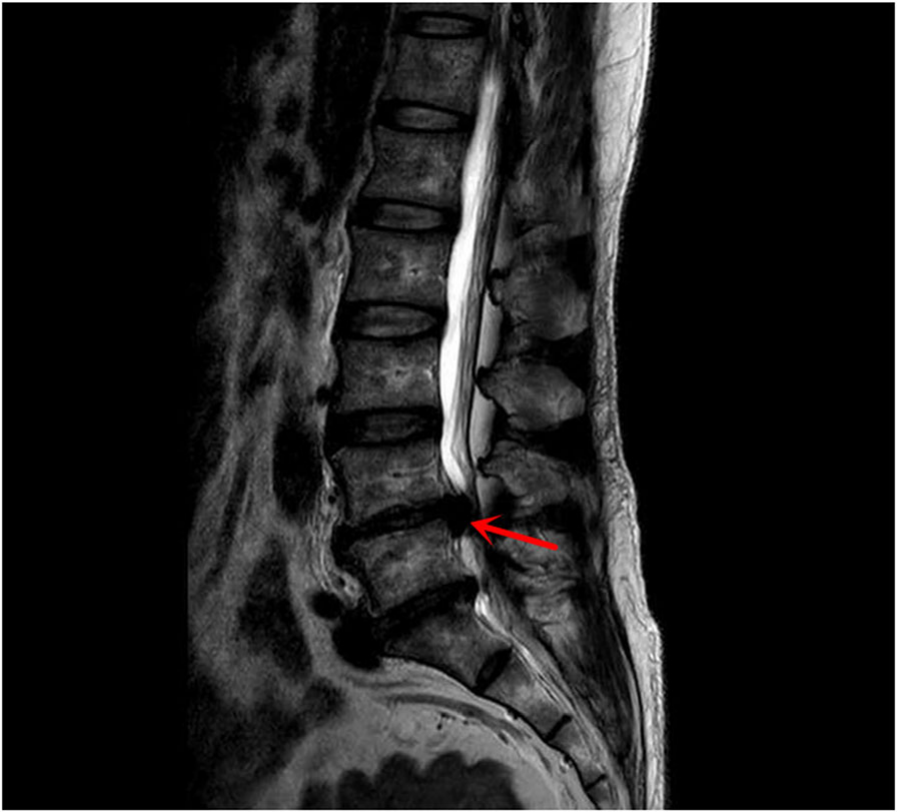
MRI imaging of the lumbar spine demonstrating a discal cyst with hypointense on T2-weighted images (red arrow)
